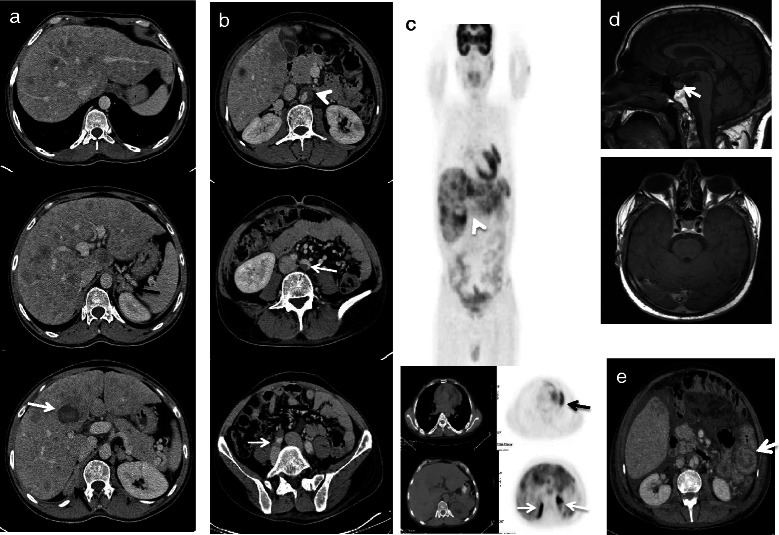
Major radiological findings. a Trans axial images of enhanced computed tomography (CT) of the abdomen showing: hypodense liver lesions in both lobes (the largest is located in segment IV adjacent to porta hepatis, white arrow), and bilateral adrenal hyperplasia (black arrows). b Aortic dissection below the level of renal arteries (white arrow head) extending to the left and right common iliac arteries (white arrows). c FDG PET-CT scan showing multiple hyper metabolic liver lesions (white arrow head), lung metastasis involving the left paracardiac area (black arrow), and diffuse bilateral hyper metabolic activity within the adrenal gland (white arrows). d Sagittal and axial (post contrast) T1 weighted MRI images showing normal pituitary gland. e Trans axial image of enhanced computed CT of the abdomen showing jeujenal intussusception
